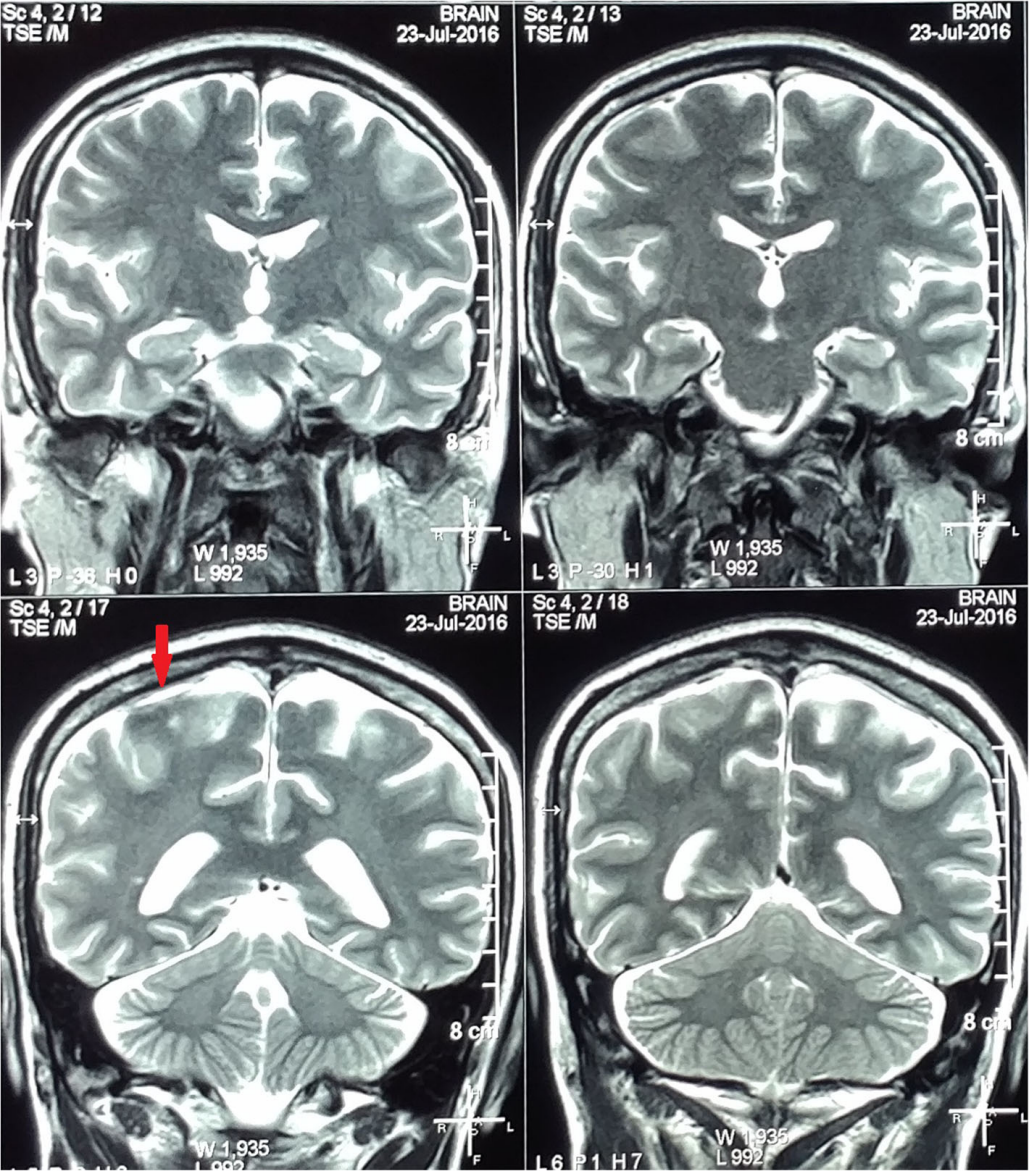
Post-treatment T2-Weighted MRI brain (coronal view) showing resolution of the subdural collection
